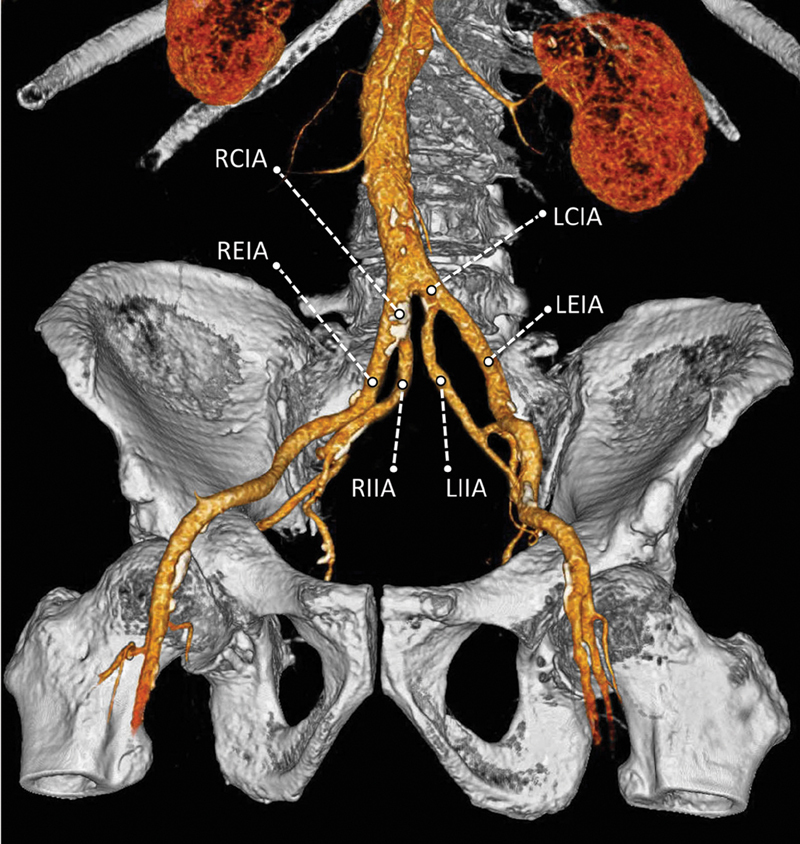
Multidetector computed tomography angiography coronal volume reconstruction demonstrating the presence of a congenital anatomical variant, with cranially displaced origin of the internal iliac arteries (right internal iliac artery [RIIA] and left internal iliac artery [LIIA]), reduced length of both common iliac arteries (right common iliac artery [RCIA] and left common iliac artery [LCIA]), and increased length of both external iliac arteries (right external iliac artery [REIA] and left external iliac artery [LEIA]).
